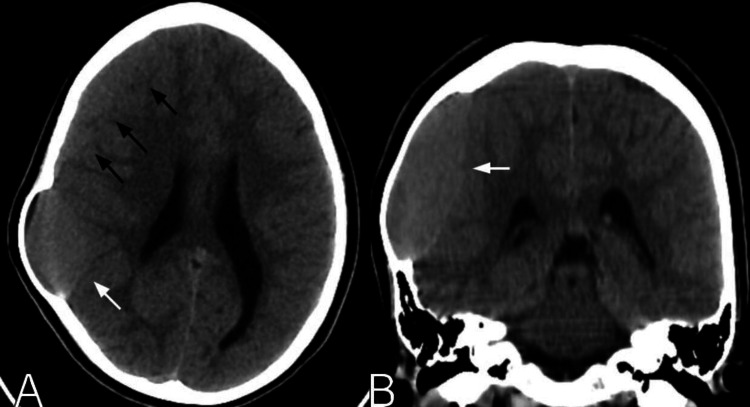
Preoperative head CT scan axial (A) and coronal (B) views showing a right isodense subacute subdural hematoma (black arrows) causing a mass effect on the underlying brain, associated with a parietal arachnoid cyst containing intracystic subacute blood (white arrows).
